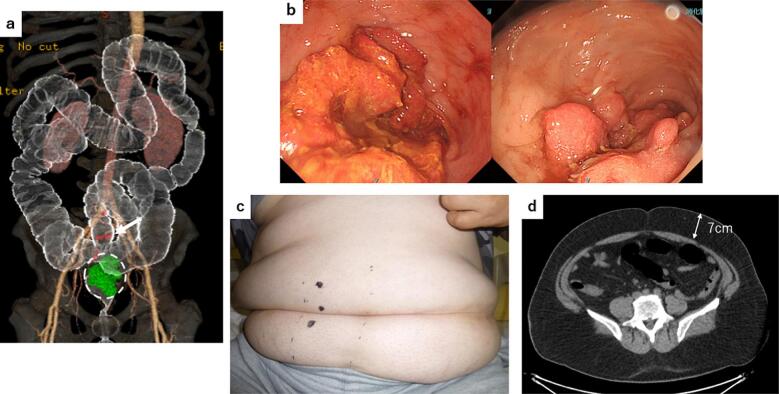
(a) In 3D-CT, the primary site of rectal cancer is indicated by a green tumor (circle), and lymph node metastases are indicated by a red node (arrow). (b) Pre-chemotherapy colonoscopy shows a semi-peripheral type 2 lesion in the rectum (left), and post-chemotherapy colonoscopy shows shrinkage from pre-chemotherapy (right). (c) Preoperative stoma site markings. (d) Subcutaneous fat thickness at the umbilical level is 7 cm. (For interpretation of the references to colour in this figure legend, the reader is referred to the web version of this article.)
